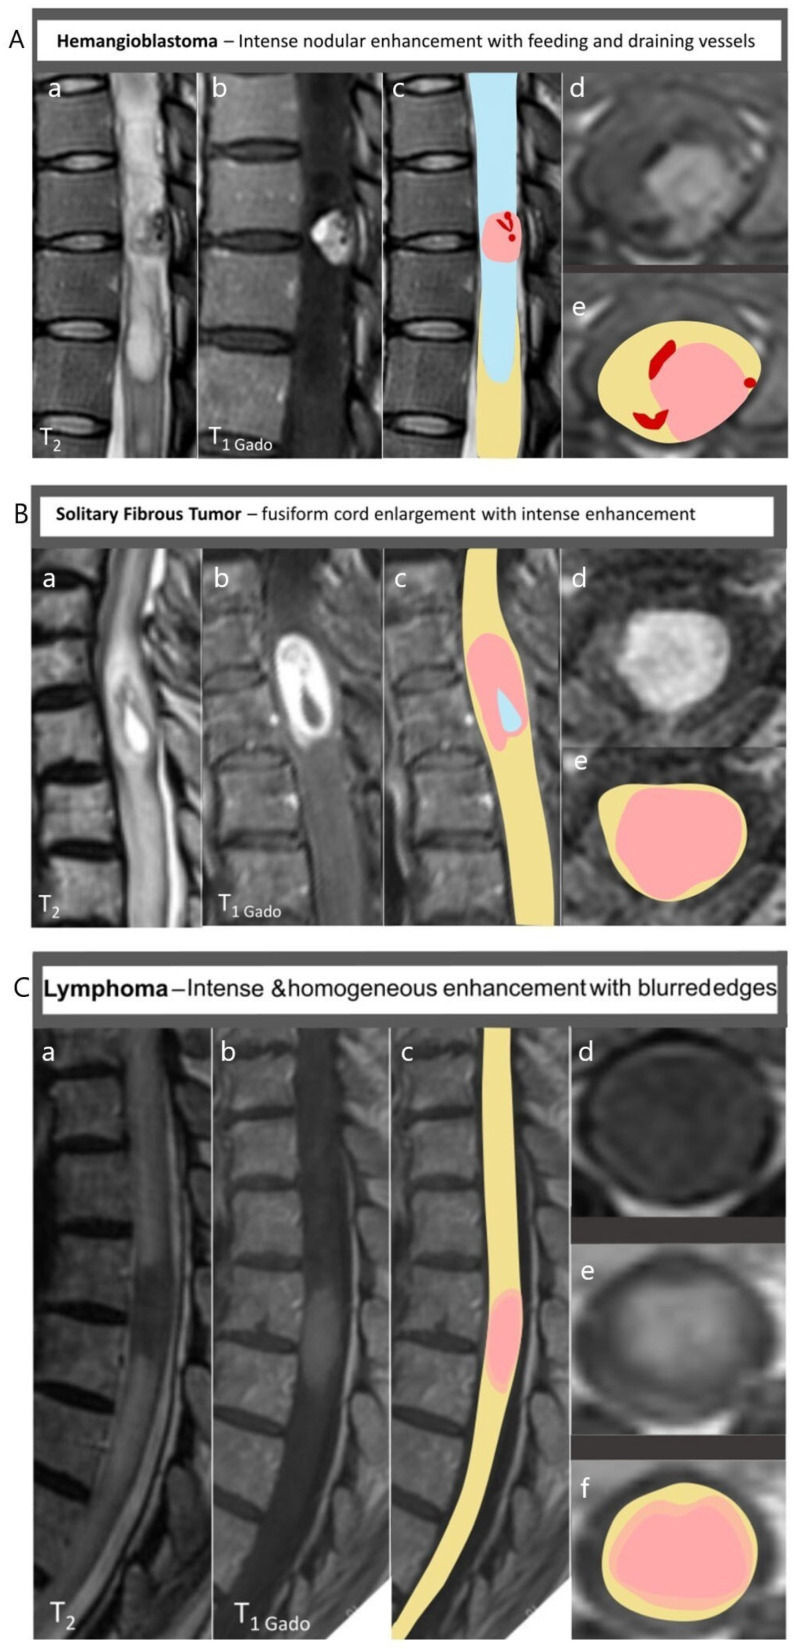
(A) Hemangioblastoma: (a) Sagittal T2-weighted image showing a hyposignal nodule with draining vessels associated to syrinx; (b) Sagittal T1-contrast-enhanced image showing an intense enhancement; (c) Sagittal pictorial representation; (d) Axial T1-contrast-enhanced image showing a nodular tumor with hyposignal draining vessels at the ventral and dorsal poles of the tumor; (e) Axial pictorial representation. (B) Solitary fibrous tumor: (a) Sagittal T2-weighted image showing a fusiform intramedullary tumor with extensive edema; (b) Sagittal T1-contrast-enhanced image showing intense enhancement; (c) Sagittal pictorial representation; (d) Axial T1-contrast-enhanced image; (e) Axial pictorial representation. (C) Lymphoma: (a) Sagittal T2-weighted image showing intramedullary hyposignal; (b) Sagittal T1-contrast-enhanced image showing homogeneous enhancement with blurred edges; (c) Sagittal pictorial representation; (d) Axial T2-weighted image; (e) Axial T1-contrast-enhanced image; (f) Axial pictorial representation.
